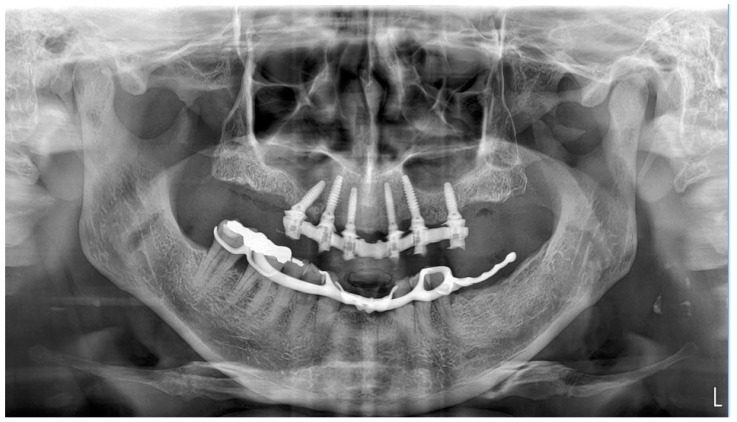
Post-surgical orthopantomography.
